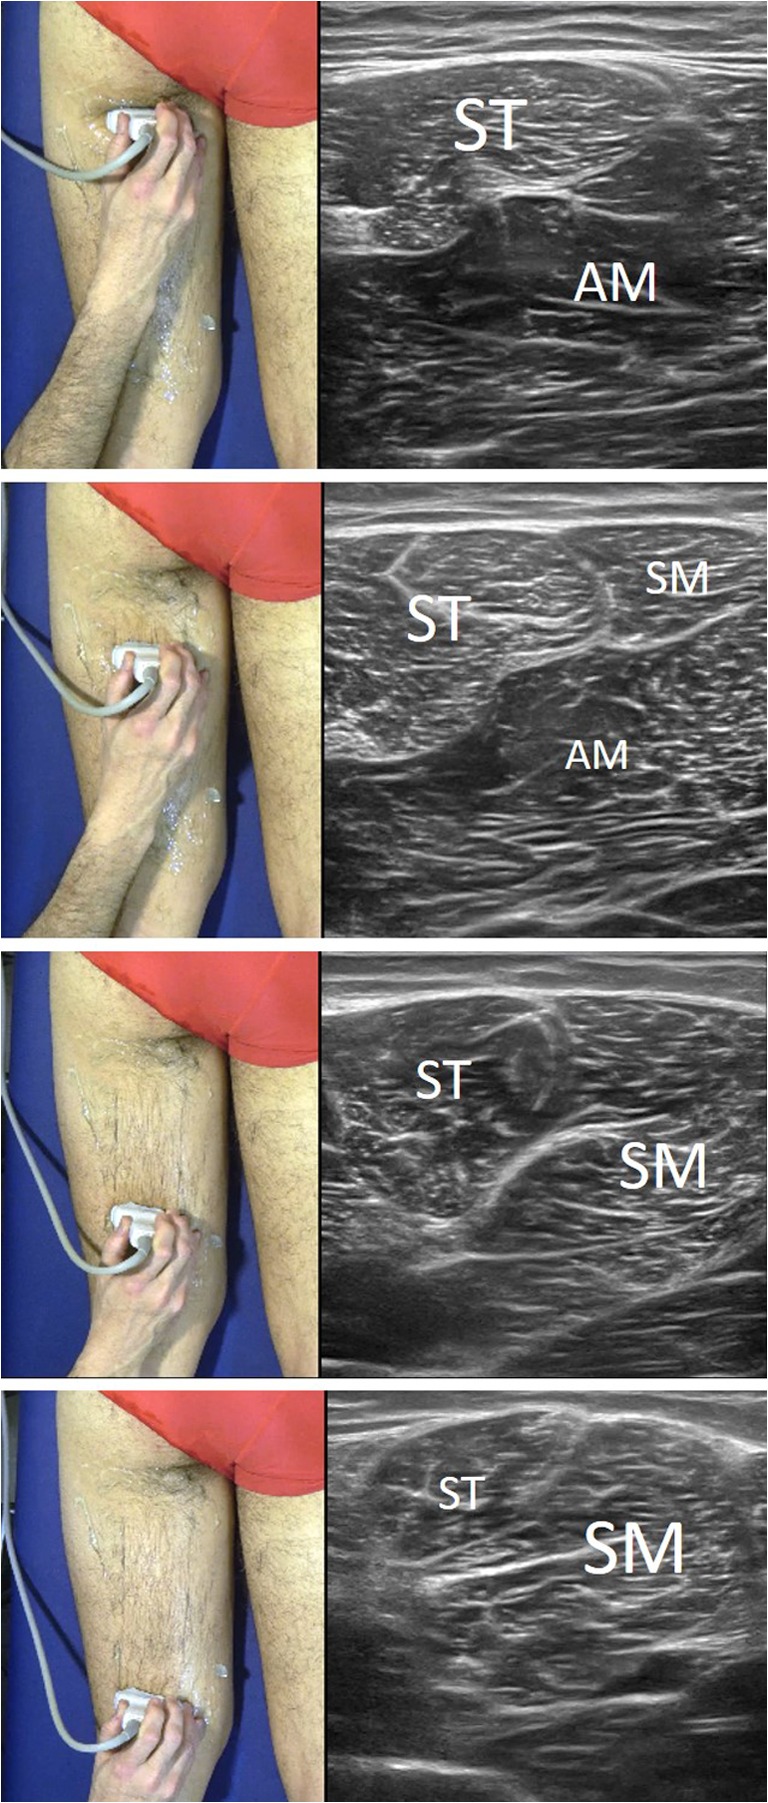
Series of short-axis ultrasound scans showing different views of the medial part of the hamstrings (medial arm of the tuning fork). As the probe is moved distally, the SM muscle mass can be seen to increase in size, while that of the ST decreases. The photographs on the left of the figure indicate probe positioning. (*ST* semitendinosus muscle, *SM* semimembranosus muscle AM: aductor magnus muscle)
